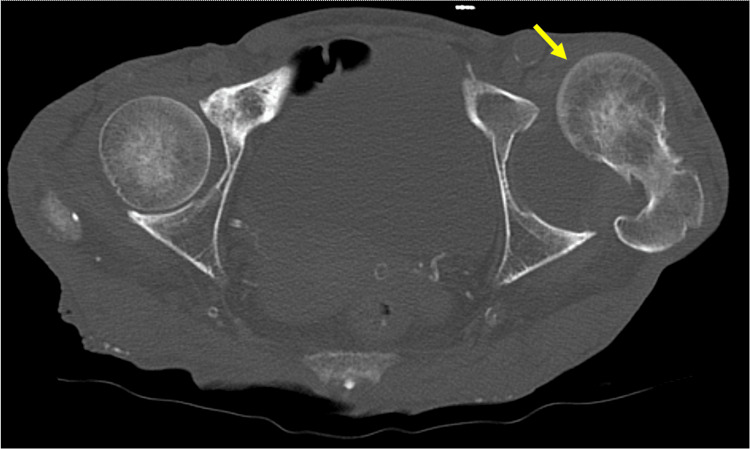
CT scan right after the reduction. The left hip joint redislocated without any external force.
